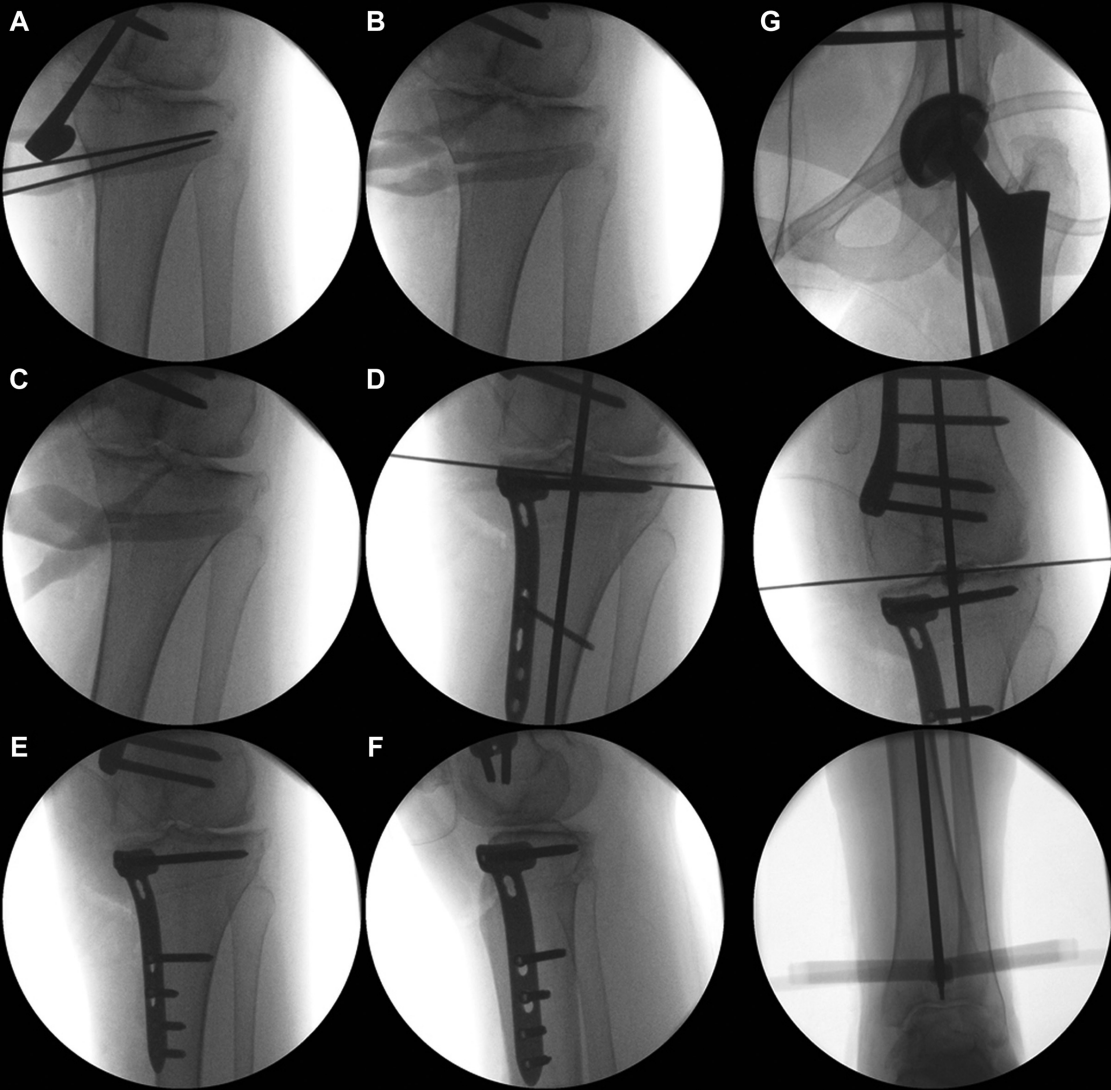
Intraoperative radiographs of the medial closing-wedge high tibial osteotomy. (A) Positioning of the K-wires aimed at the fibular head. (B, C) Removal of the bone wedge and closing of the osteotomy. Distal to the osteotomy a bicortical screw is placed via eccentric drilling. (D) After compression of the osteotomy the alignment is checked radiographically using an alignment rod. (E, F) Completed high tibial osteotomy. (G) Alignment control using the alignment rod.
